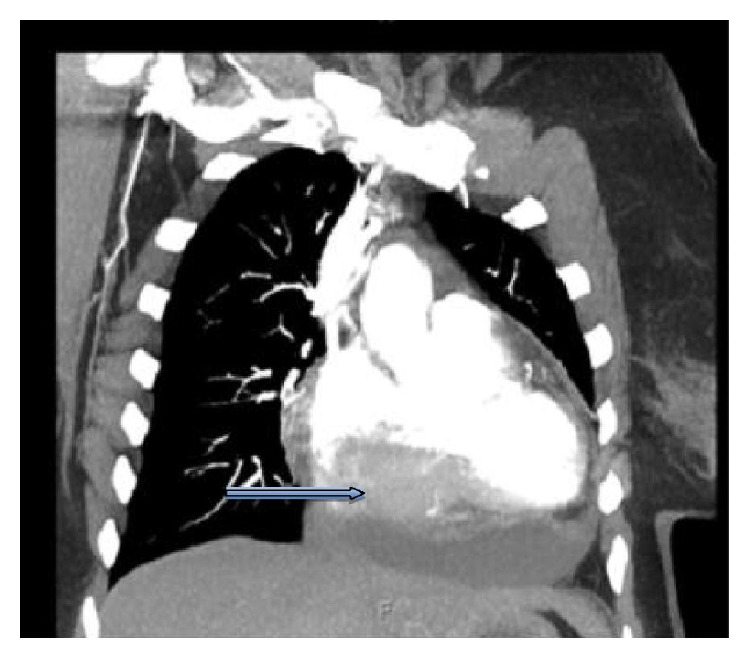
Large right cardiac mass (arrow) on CT angiography.
